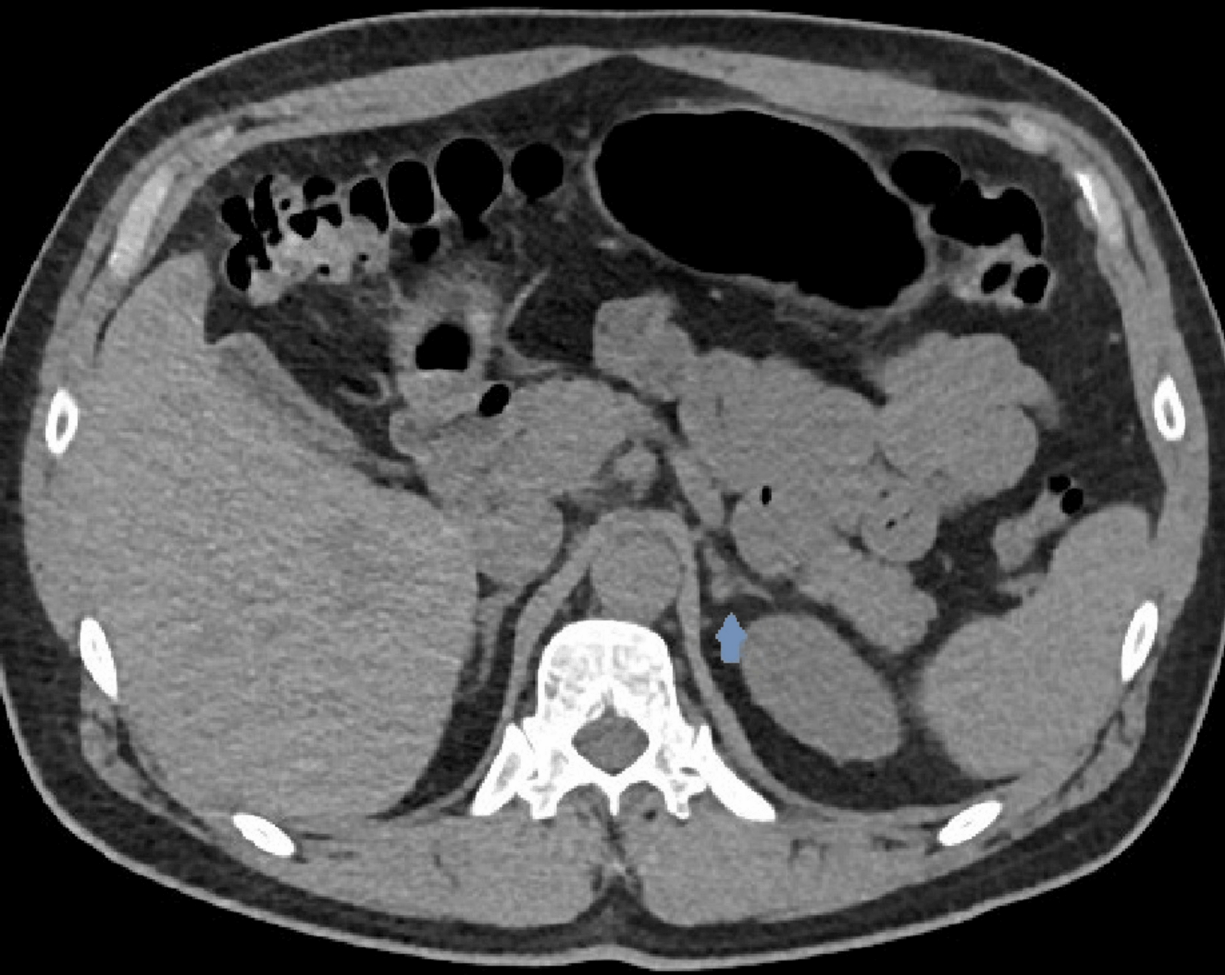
The arrow shows a mildly nodular left adrenal gland on an adrenal CT scan (axial) but without any discrete nodule.
